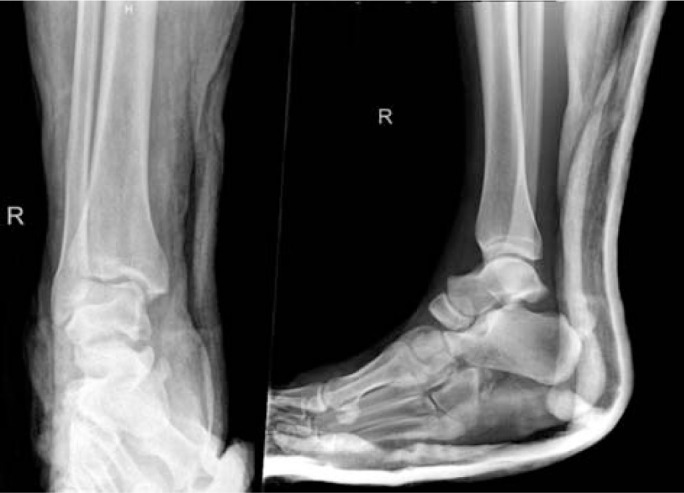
Anteroposterior and lateral ankle radiographs showing dislocations of the subtalar, calcaneocuboid and naviculocuneiform joints.
